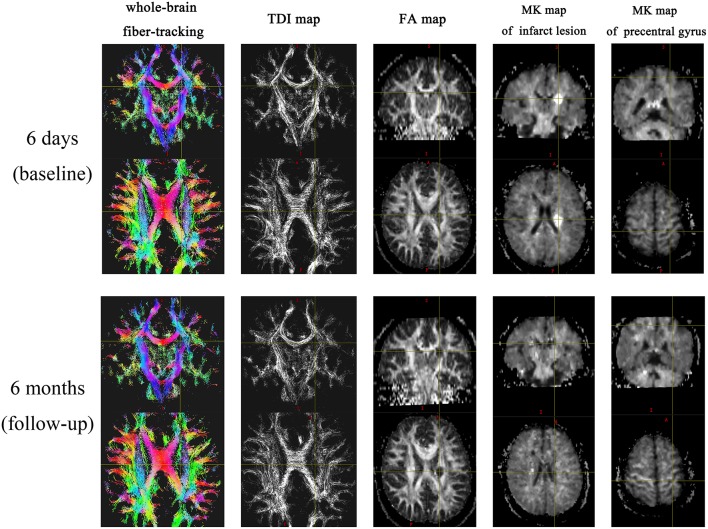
Magnetic resonance images from a representative patient.
